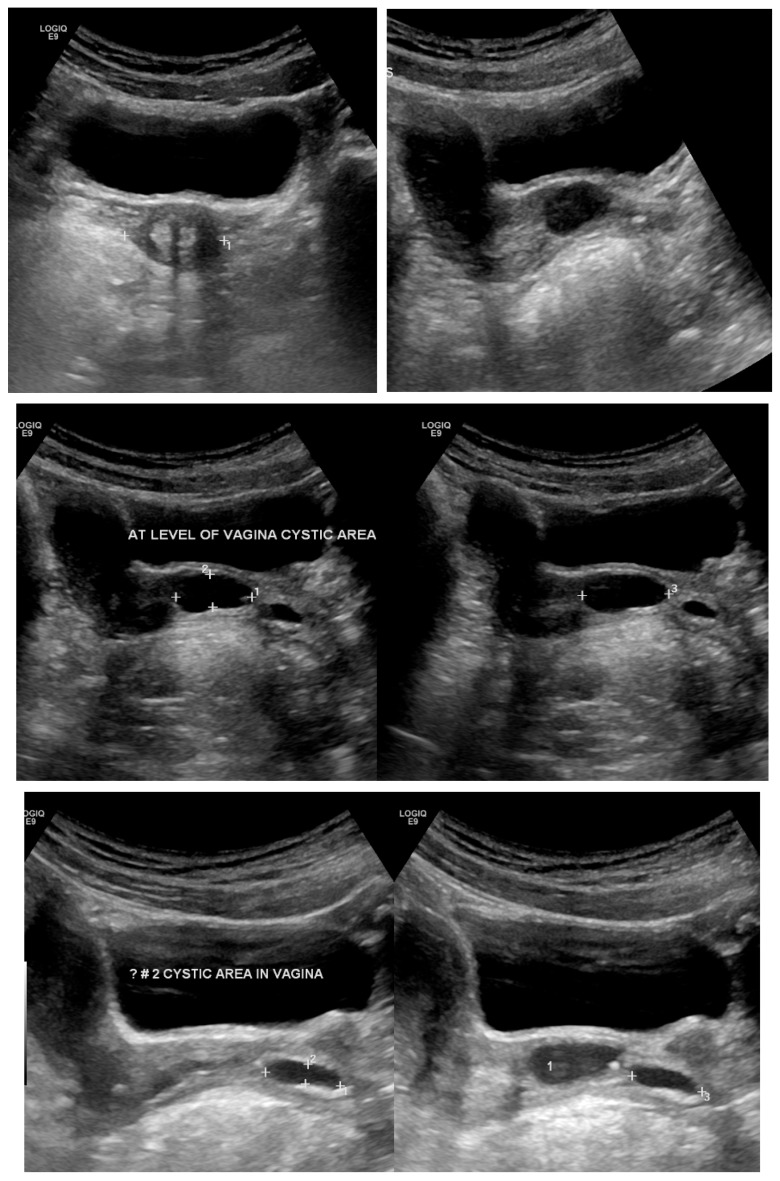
Follow up Transabdominal pelvic ultrasound in Case 4 demonstrating a lower vaginal cystic structure communicating with an ectopic/dysplastic left ureter, associated with congenital absence of the left kidney.
